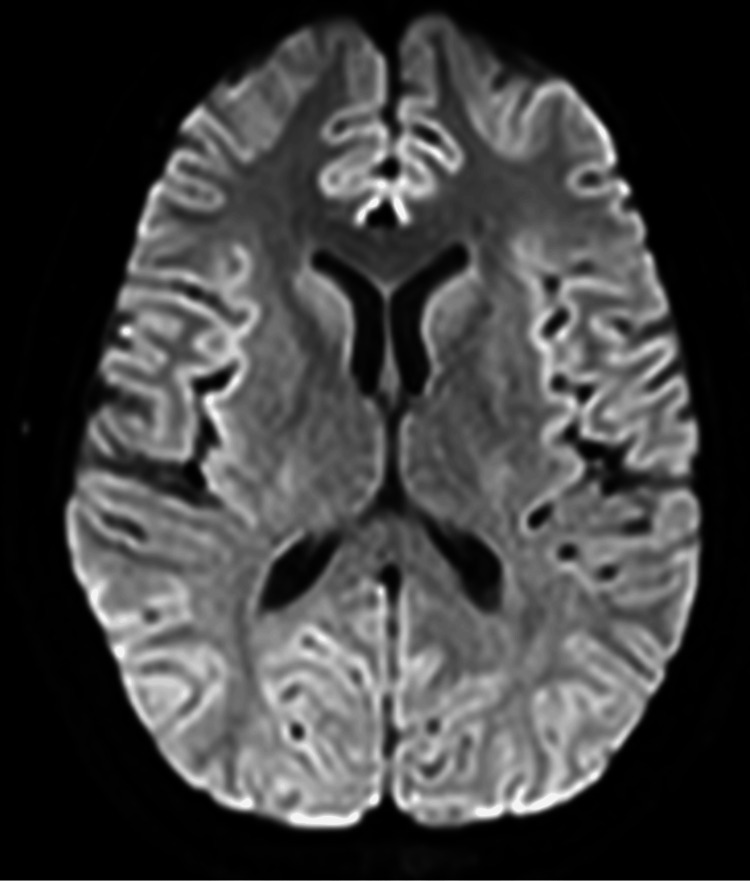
Axial brain MRI, diffusion-weighted imaging sequence, without diffusion restriction
